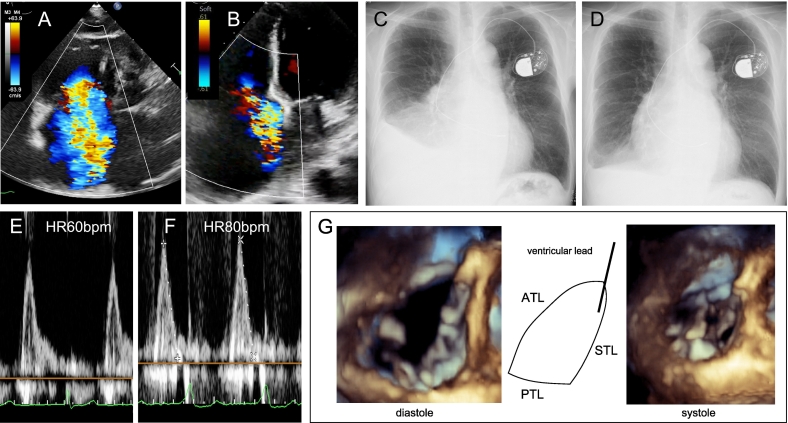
This figure demonstrates the longitudinal changes in tricuspid regurgitation and pleural effusion before and after heart rate optimization. (A) Massive tricuspid regurgitation before rate adjustment. (B) Moderate-to-severe tricuspid regurgitation after 5 months at 80 bpm. (C) Chest X-ray showing bilateral pleural effusion before rate adjustment. (D) Chest X-ray after 5 months showing resolution of pleural effusion. (E) Transmitral flow at baseline, showing E wave ending before QRS onset. (F) Transmitral flow after pacing at 80 bpm, also preserving E wave before QRS. (G) 3D transthoracic echocardiography confirming no interference of the pacemaker lead with the tricuspid valve. ATL, anterior tricuspid leaflet; STL, septal tricuspid leaflet; PTL, posterior tricuspid leaflet.
